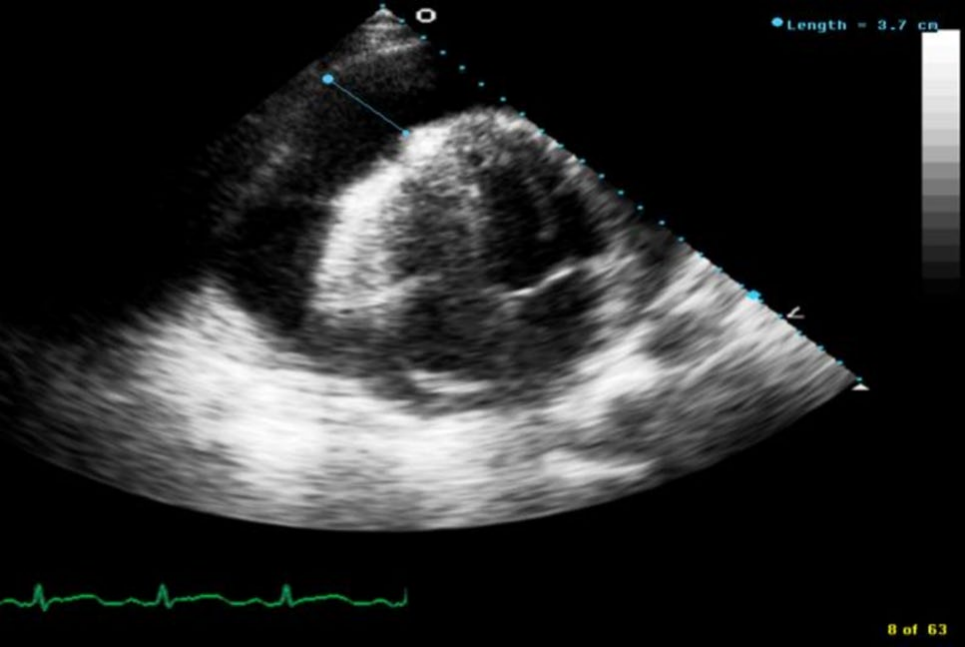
Transthoracic echocardiogram (TTE) in an apical four-chamber view demonstrating a large circumferential pericardial effusion with maximum diameter of 37 mm
